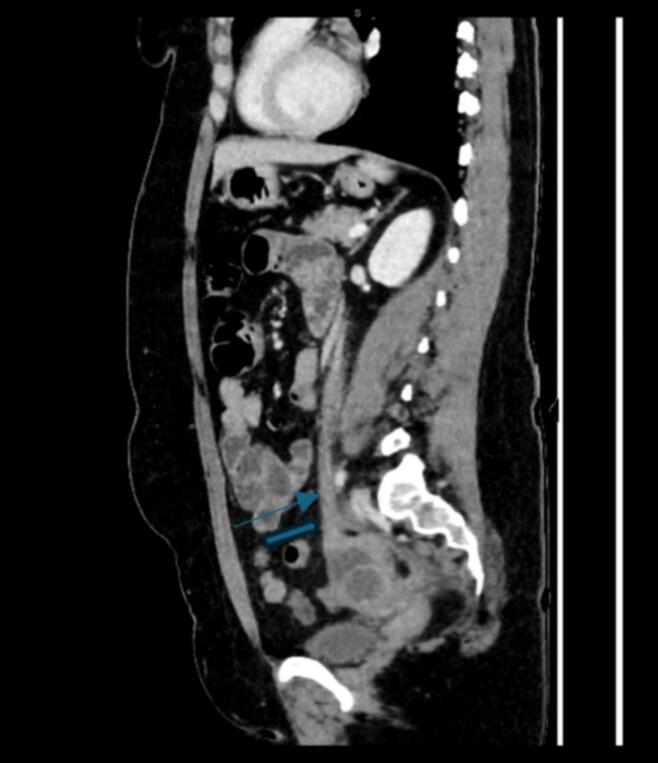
Sagittal reconstructed contrast-enhanced CT image demonstrating poorly enhancing left ovarian vein (thin arrow), and partially seen left ovarian cyst (thick arrow).
